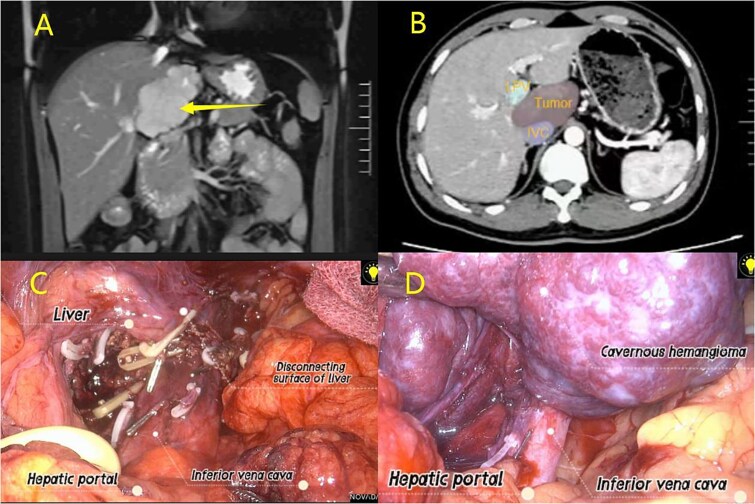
(A) MRI examination; (B) CT examination; (C–D) Laparoscopic resection of caudate lobe hemangioma procedure.
